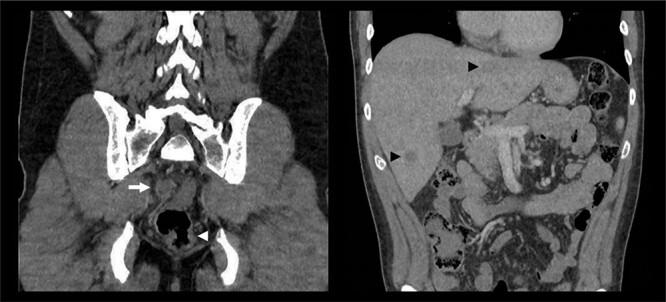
CT abdomen-pelvis with intravenous contrast demonstrating rectal wall irregularity (white arrowhead), bulky mesorectal lymphadenopathy (white arrow) and multiple hypoattenuating liver lesions (black arrow heads).
